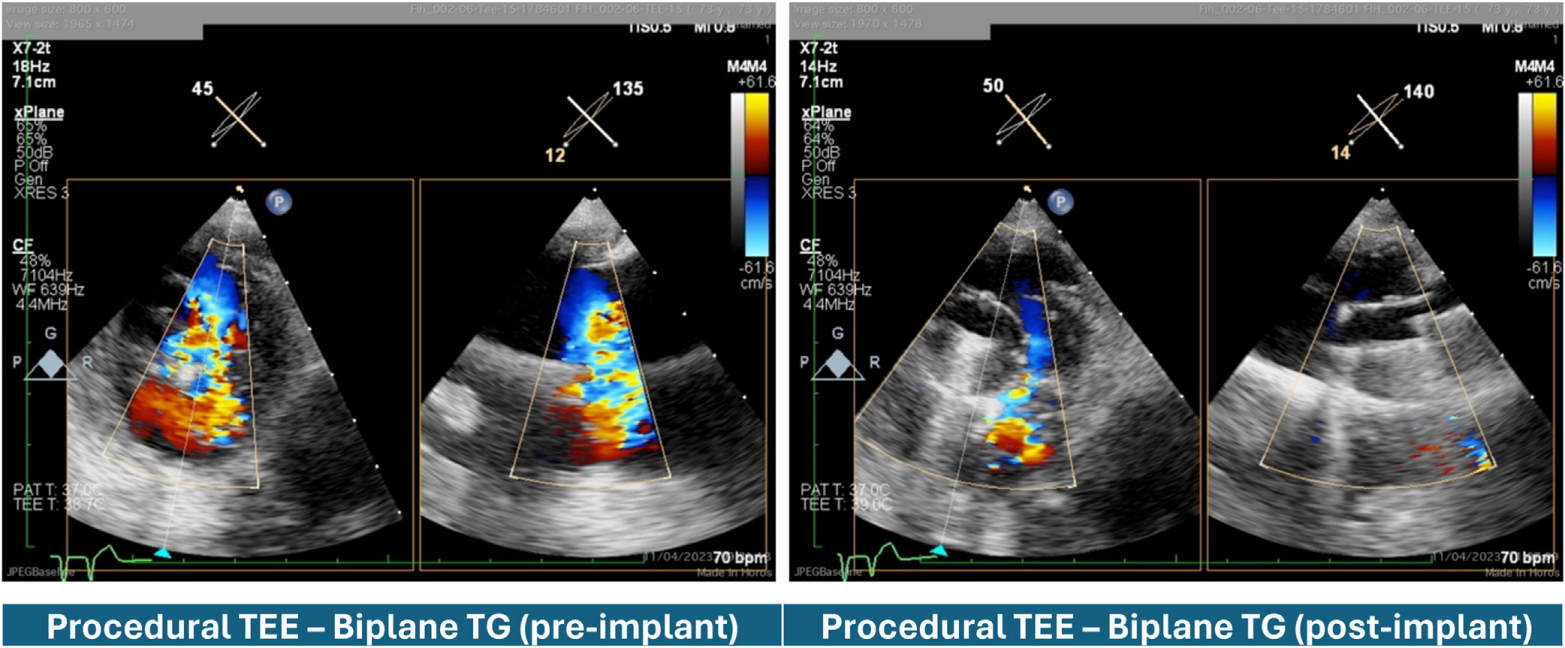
Biplane procedural TEE views. On the left transgastric short and long axis view of baseline TR demonstrating the broad central TR jet with the central pacemaker lead. On the right, a similar transgastric bi-plane view after device implantation demonstrating the coaptation valve filling the majority of the regurgitant orifice with mild to moderate residual TR in the anteroseptal commissure and deflection of the lead anteriorly. Abbreviations: 2D, 2-dimensional; bpm, beats per minute; CF, color flow; TEE, transesophageal echocardiography; TG, transgastric; TR, tricuspid regurgitation.
